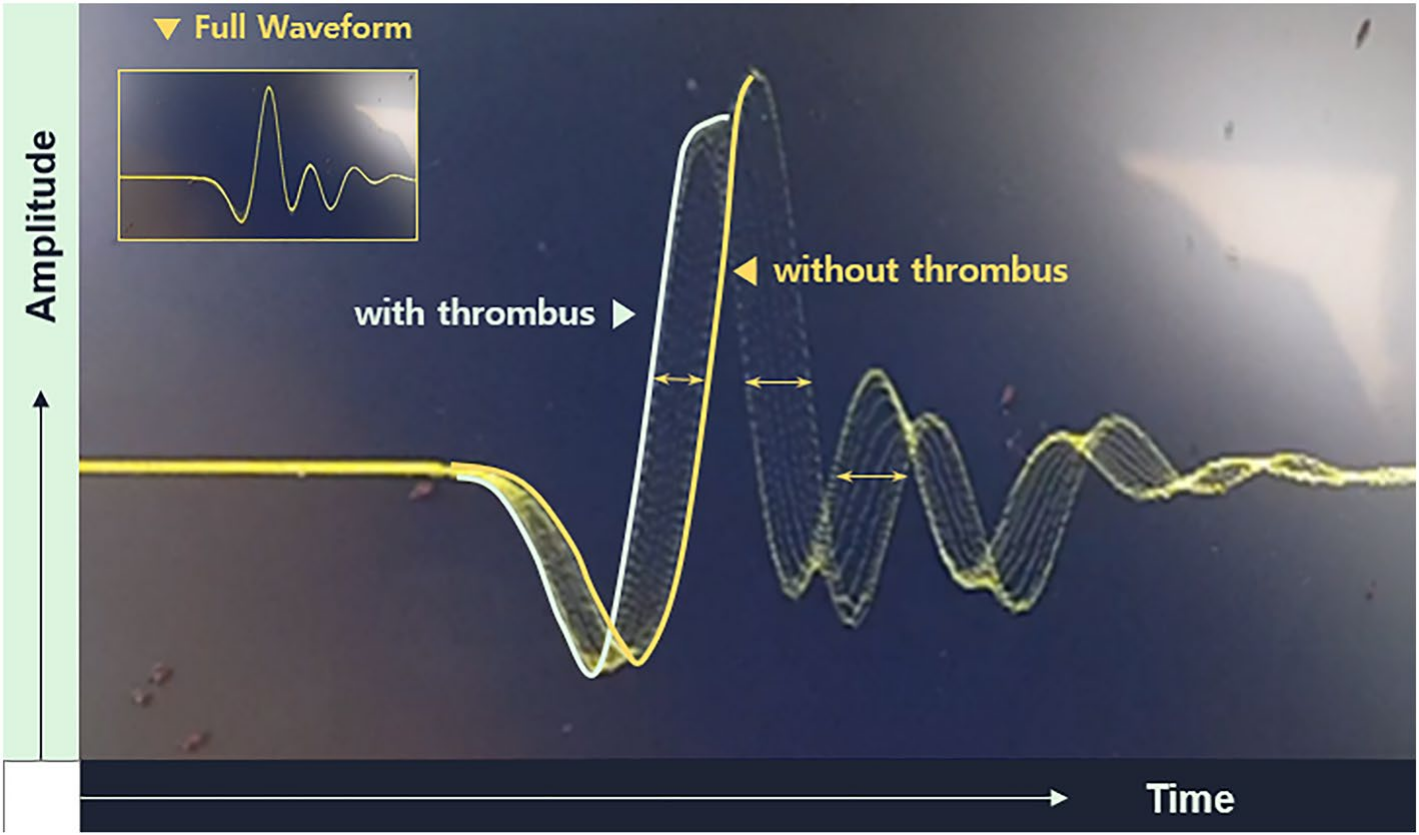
Ultrasonic signal at the DSO. Ultrasonic signal from the blood flowing in the bloodline without thrombi. The ultrasonic signal from the blood flowing through the bloodline containing a thrombus (at the flow of 1.5 LPM).
